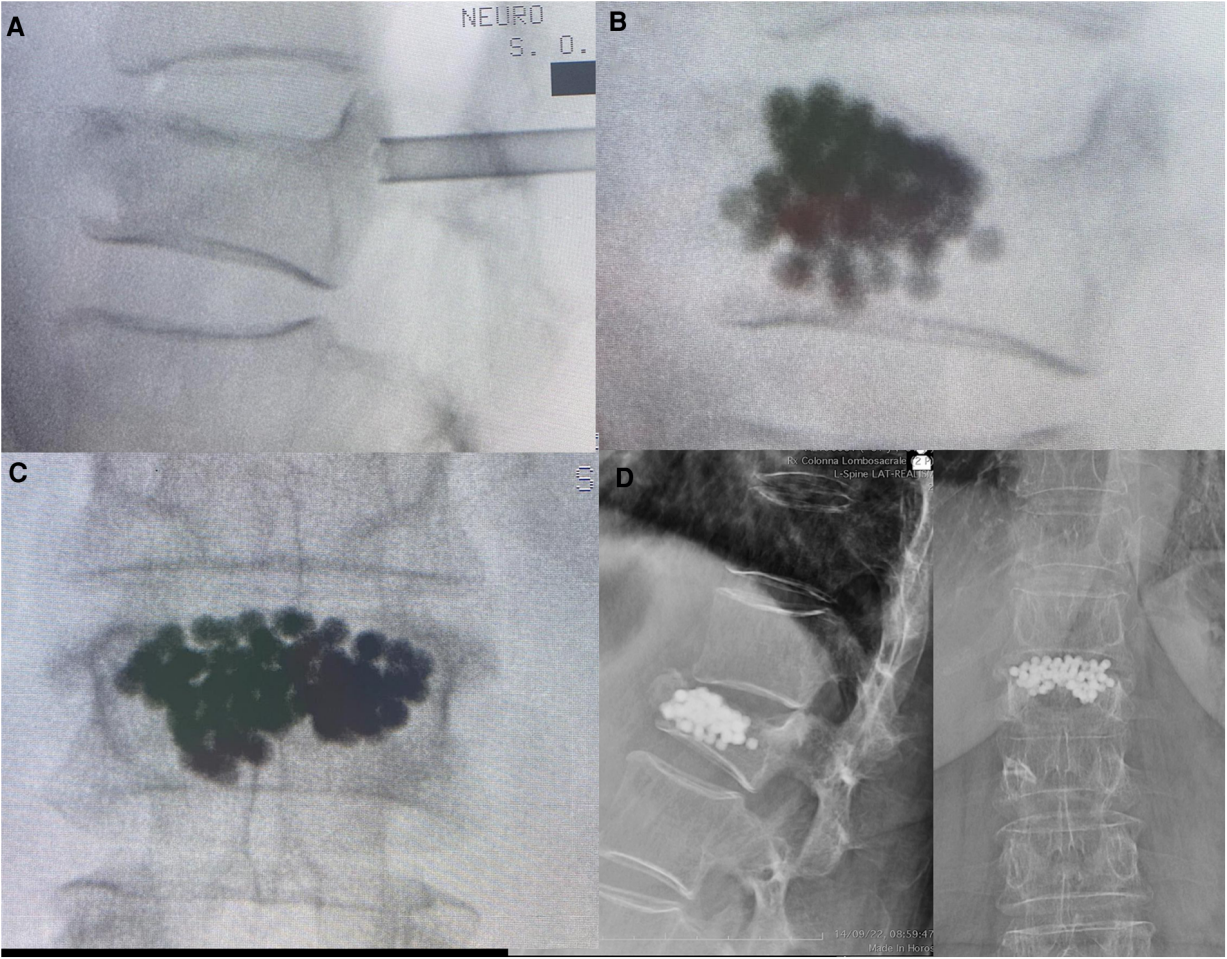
A representative case of an 82-year-old woman with vertebral osteoporotic compression L1 fracture without posterior wall involvement, unresponsive to pain drugs. (A) Cannula insertion and (B,C) intraoperative view after the titanium microsphere injection. (D) Radiographic follow-up control at 3 months with signs of partial ossification and fusion.
